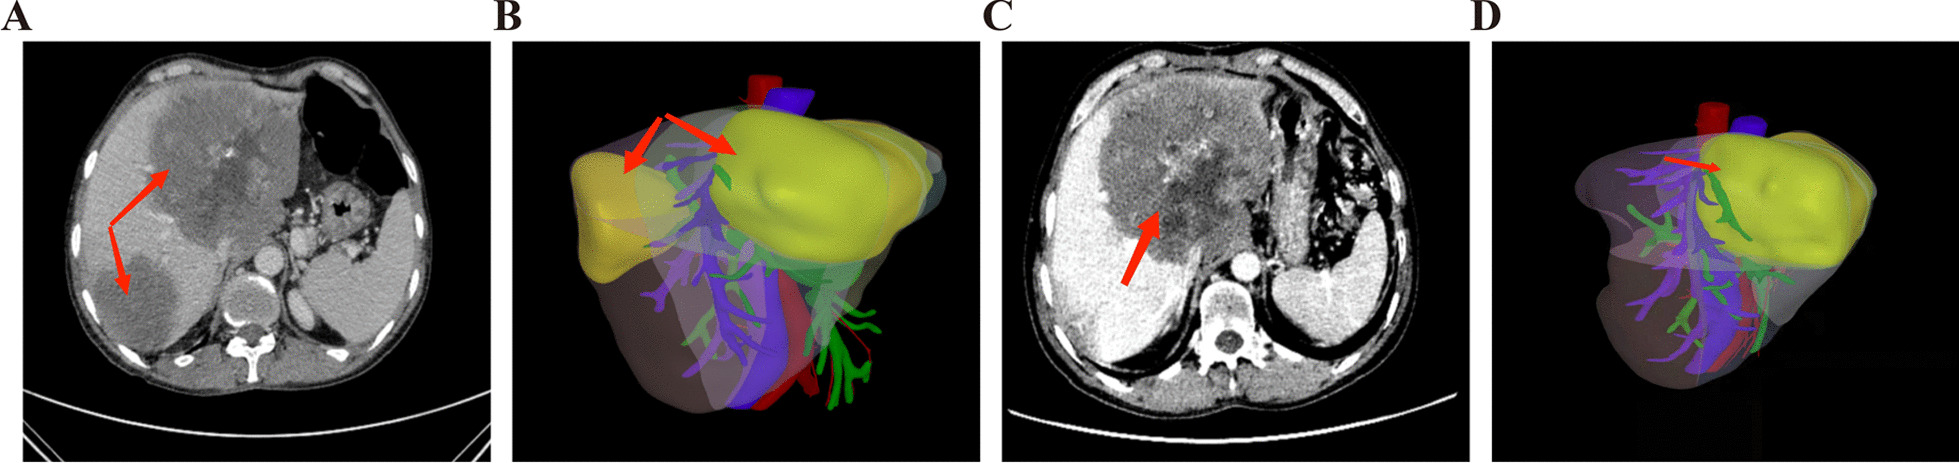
After TSH procedures, TSH3 patient, with FLRV/TLV > 30%, was able to receive ELRA procedures. A the first stage preoperative CT image; B the first stage preoperative digital 3D reconstruction of liver (FLRV/TLV < 30%); C CT image before the second stage operation; D The second stage preoperative digital 3D reconstruction of liver (FLRV/TLV > 30%)
